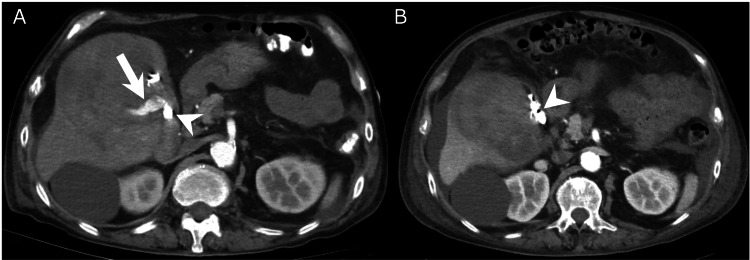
Contrast-enhanced CT image (A) An early phase contrast-enhanced CT image two days after the first transarterial embolization reveals residual extravasation in the gallbladder bed (white arrow). The n-butyl-2-cyanoacrylate cast in the pseudoaneurysm is also demonstrated (white arrowhead). (B) The follow-up contrast-enhanced CT 11 days after the second transarterial embolization demonstrates the n-butyl-2-cyanoacrylate cast in the gallbladder (white arrowhead) and no extravasation. There are no findings suggestive of ischemic cholecystitis. CT, computed tomography
